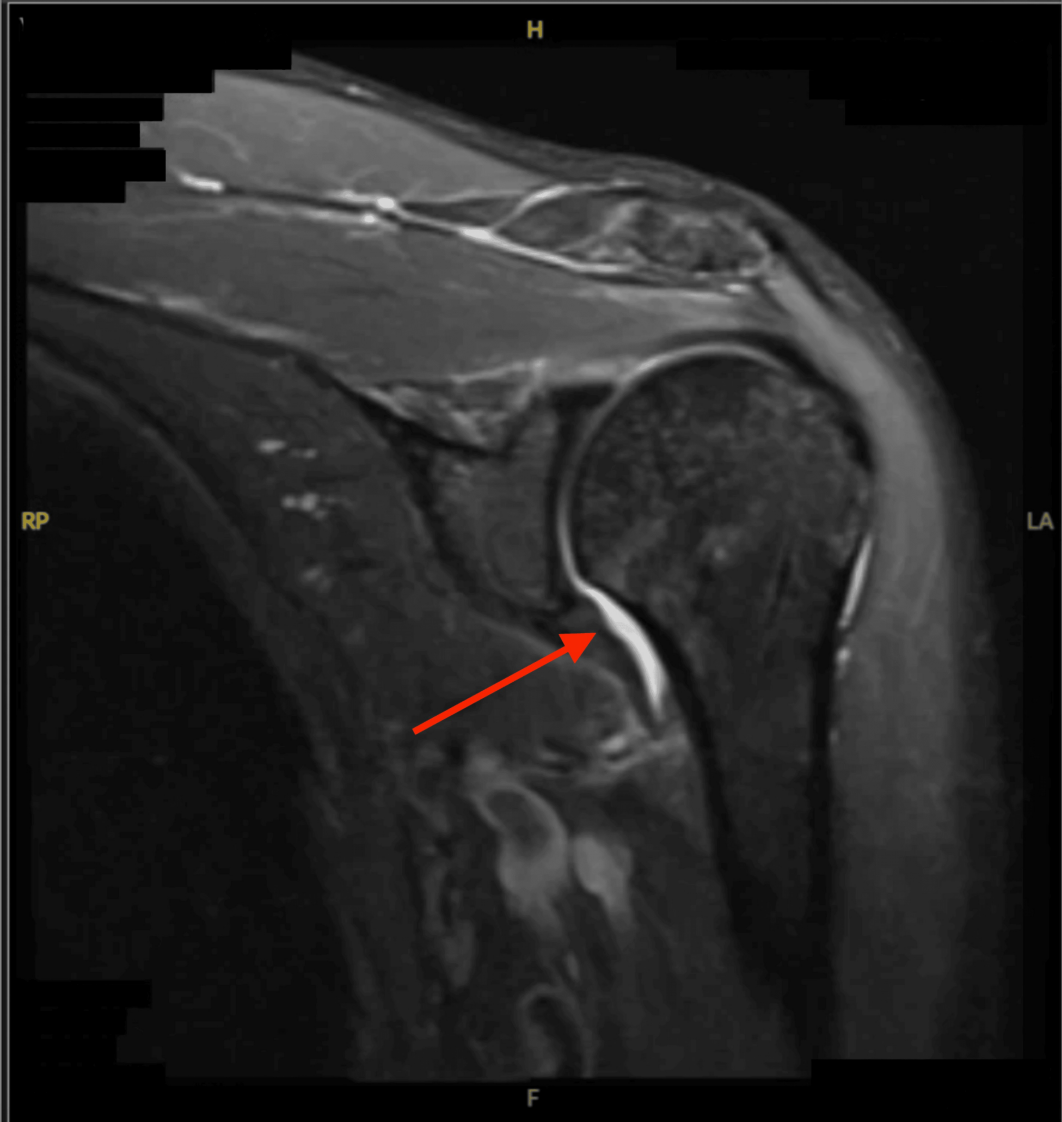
Sagittal T2-weighted MRI of the left shoulder demonstrating supraspinatus tendinosis with fluid signal in the subacromial space and capsular changes consistent with adhesive capsulitis MRI: magnetic resonance imaging; H: head; F: feet; LA: left anterior; RP: right posterior
